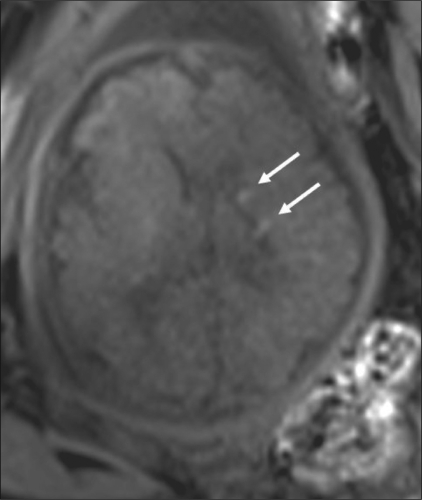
Single live gestation at 30 weeks. T1W axial MRI image at the level of the lateral ventricles shows tubers as subependymal hyperintense nodules (arrows).
